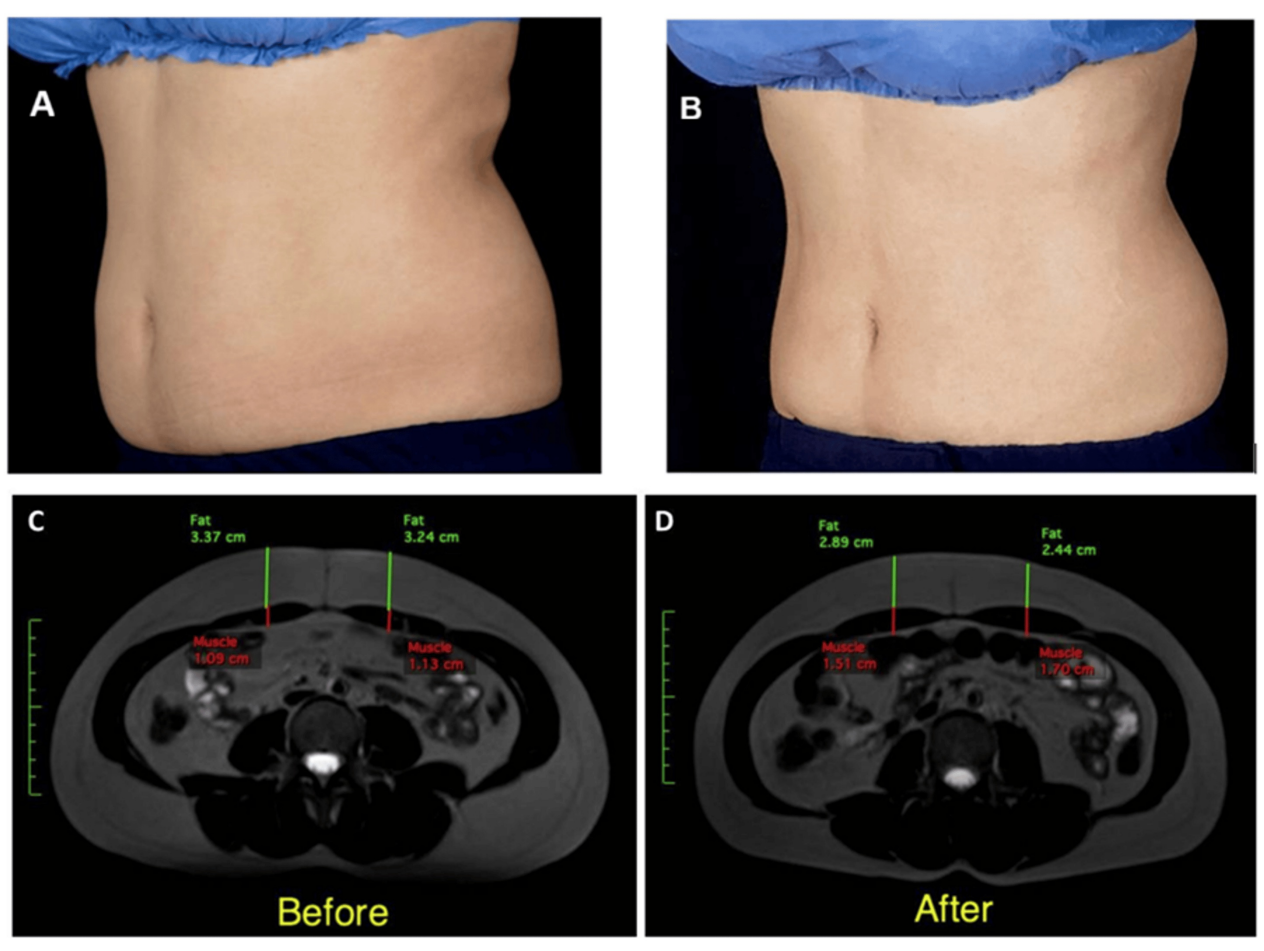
(A) Abdominal photograph of patient No. 9 before treatment, (B) After the treatment, (C) Abdominal MRI Scan of patient No. 9 before treatment, (D) After the treatment
